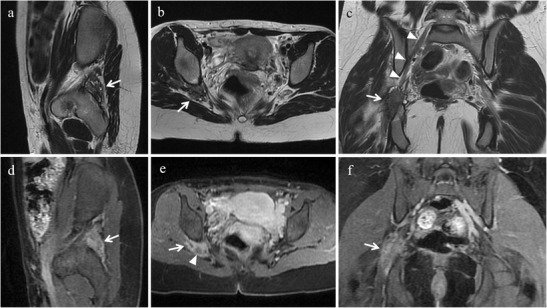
Extrapelvic sciatic nerve endometriosis in a 31-year-old woman who has been suffering from cyclic sciatica for about 2 years. (a) Sagittal, (b) axial and (c) coronal T2-weighted images show hypointense spiculated soft-tissue thickening centred around the right sciatic nerve at the sciatic notch (white arrows). (d) Sagittal, (e) axial and (f) coronal contrast-enhanced fat-suppressed T1-weighted images display enhancement of the mass (white arrows). Note the sciatic nerve cephalad to the lesion (white arrowheads in c) and within the lesion (white arrowhead in e)
